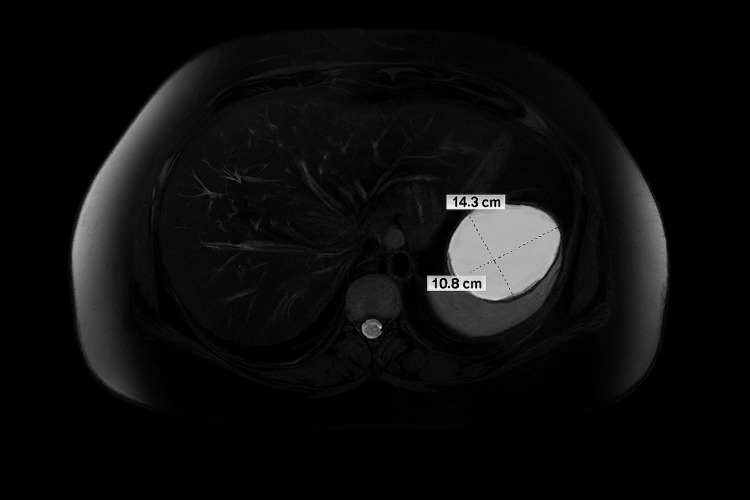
Axial T2-weighted MRI image of the upper abdomen showing a large, well-defined, unilocular exophytic splenic lesion measuring approximately 6.1 × 7.1 cm The lesion demonstrates high signal intensity with an internal fluid-fluid level and a thick wall of low T2 signal intensity, suggestive of a complex cystic lesion (possible splenic pseudocyst or epithelial cyst).
